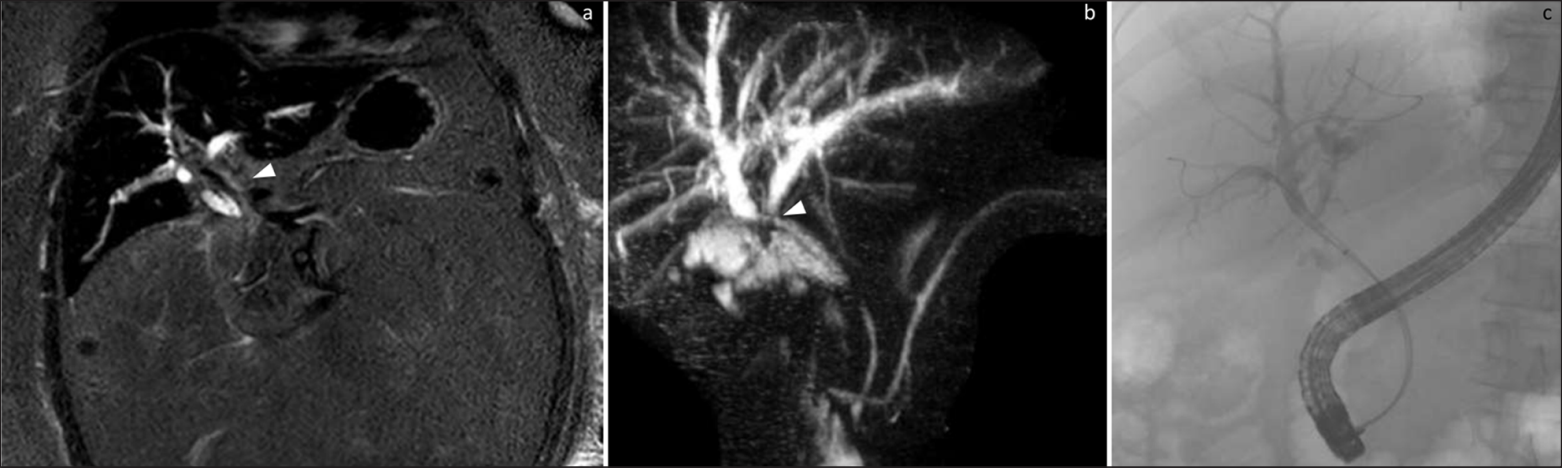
During the ERCP, biliary sphincterotomy and hilar stricturoplasty were performed, the right main hepatic duct was dilated with a 4mm balloon, and a plastic stent was placed. Four days after the procedure, the patient developed severe left upper quadrant abdominal pain, hypotension, altered mental status, and anemia. An urgent contrast-enhanced computed tomography (CT) of the abdomen (Figure 2) revealed a subcapsular splenic hematoma with active extravasation of contrast (black arrow), and moderate-sized hemoperitoneum predominantly along the spleen and extending into the left paracolic gutter and pelvis.
